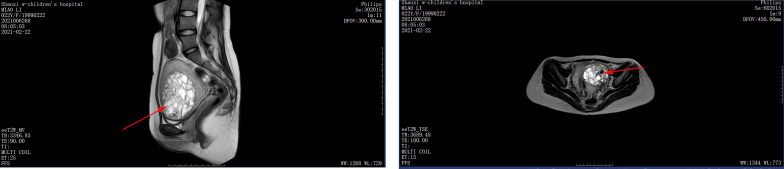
Pelvic MRI. Twenty-one days after the second molar evacuation, pelvic MRI scan showing a 7.1 × 6.1 × 8.2 cm mass invading the left anterior uterine wall, extending from the muscular layer to the serosal layer.
